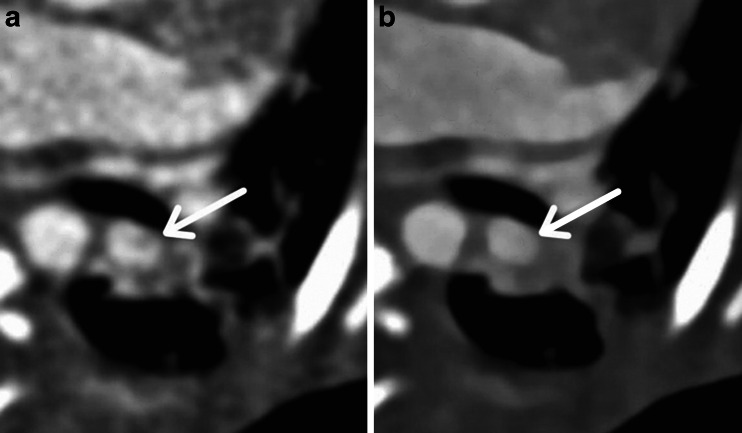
Contrast-enhanced CT transverse images of a 35-day-old girl. Example images of image noise in the left pulmonary artery. a CT image of 70-kV (S0, pure filtered back projection). There was image noise (Grade 2) in the left pulmonary artery (*arrow*), which might be confused with embolism. b CT image of 70-kV (S5, high-strength sinogram-affirmed iterative reconstruction). There was no image noise in the left pulmonary artery (*arrow*)
